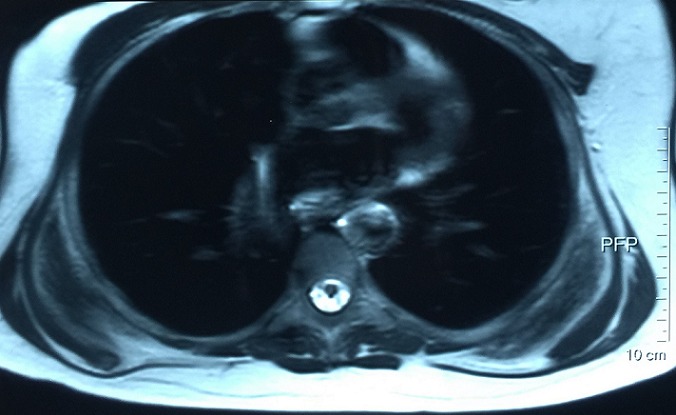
MRI image of bilateral elastofibroma dorsi (transversal view)
